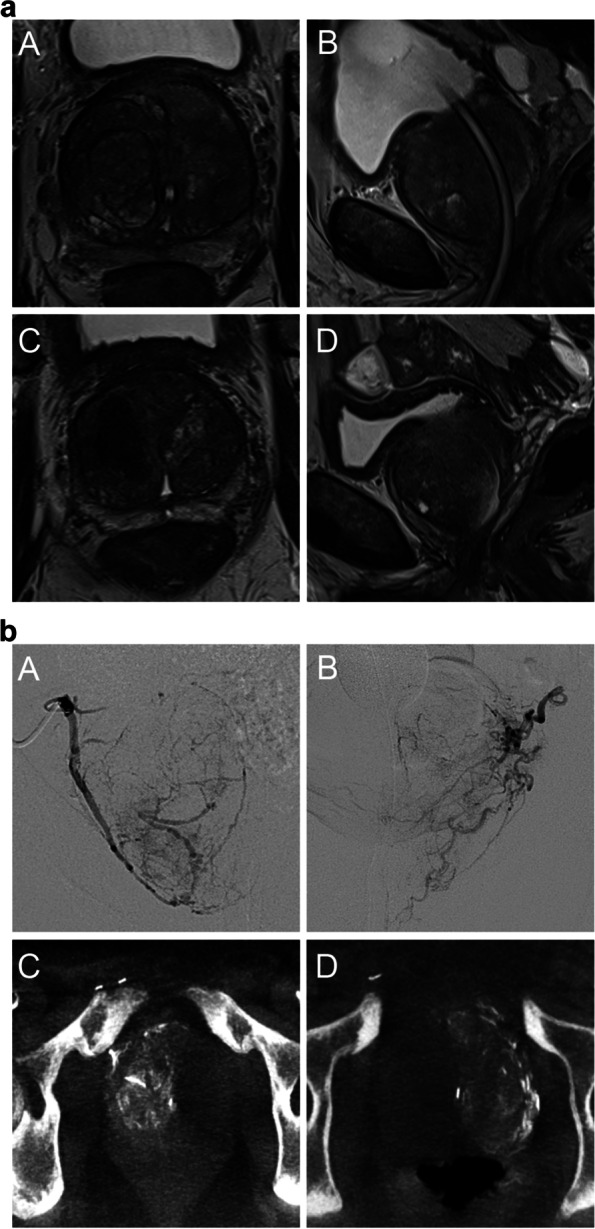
a Wasserman type 1, modified BPH classification 1 (preurethral); A: T2-weighted axial baseline B: T2-weighted sagittal baseline; compression and displacement of the urethra posteriorly; C: T2-weighted axial follow-up D: T2-weighted sagittal follow-up. Volume reduction of 130 ml to 76 ml (42%); IPSS 10 to 2 (80%). b A/B: Digital subtraction angiography from right/left prostatic artery; C/D cone-beam-computed tomography with contrast injection from right/left prostatic artery
